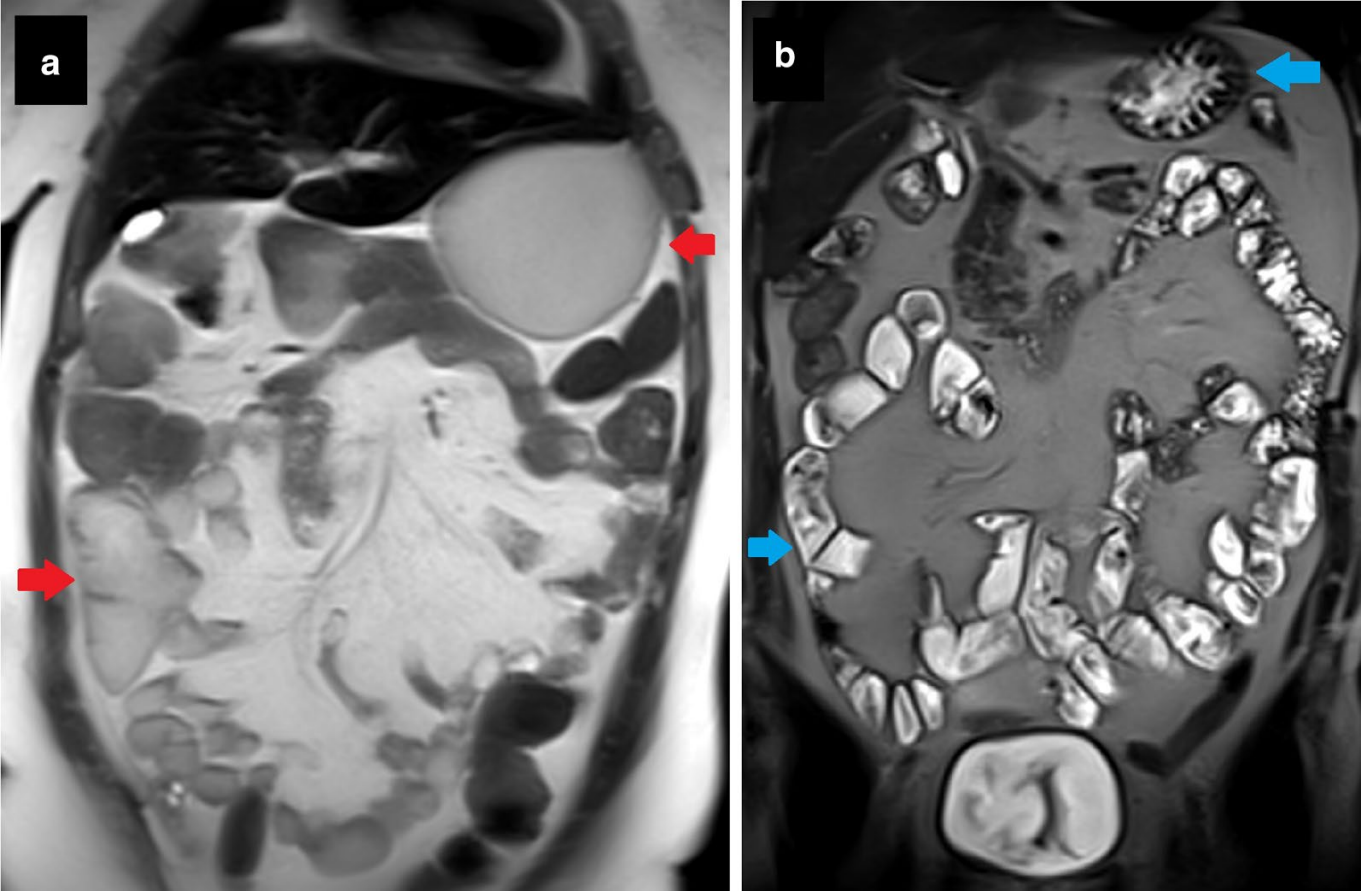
A technique error causing unnecessary effort. A 47-year-old female patient was investigated for inflammatory bowel disease, and MRI enterography was ordered. Before the procedure, the patient had drunk the gadolinium-containing contrast agent in the radiology department by mistake. Due to the paramagnetic feature of gadolinium, the required image quality for evaluation of the intestinal lumen could not be achieved. The MRI technician realized the problem and immediately informed the radiologist. After the radiologist’s evaluation, the study was rescheduled. Although the technical error caused unnecessary effort (repeated examination) in this case, the effective communication between the radiologist and MRI technician enabled them to recognize and correct the error earlier (red arrows, a intestinal lumen with gadolinium, blue arrows, b intestinal lumen with mannitol in another patient)
